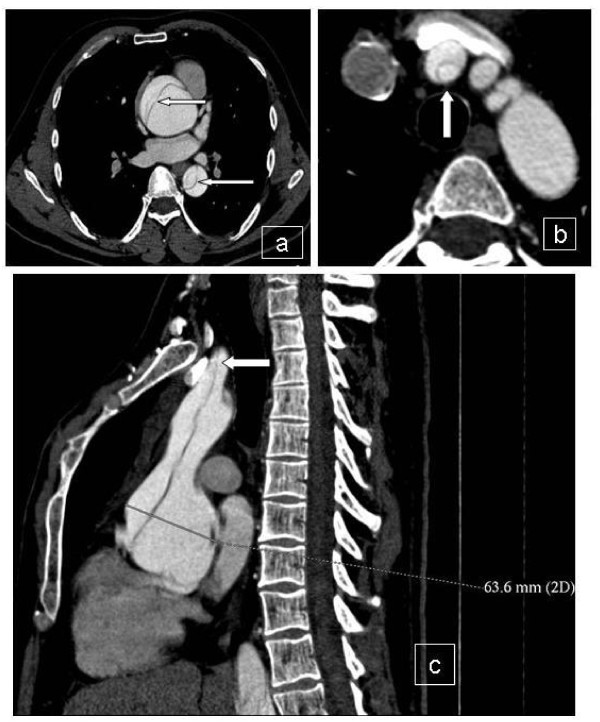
CT scan with contrast reveals ascending aorta dilatation with intimal flap (arrows) in the ascending and descending aorta (a). Innominate artery dissection (b) and reconstructed image showing aortic root dilatation, together with aorta and innominate artery dissection (c).
